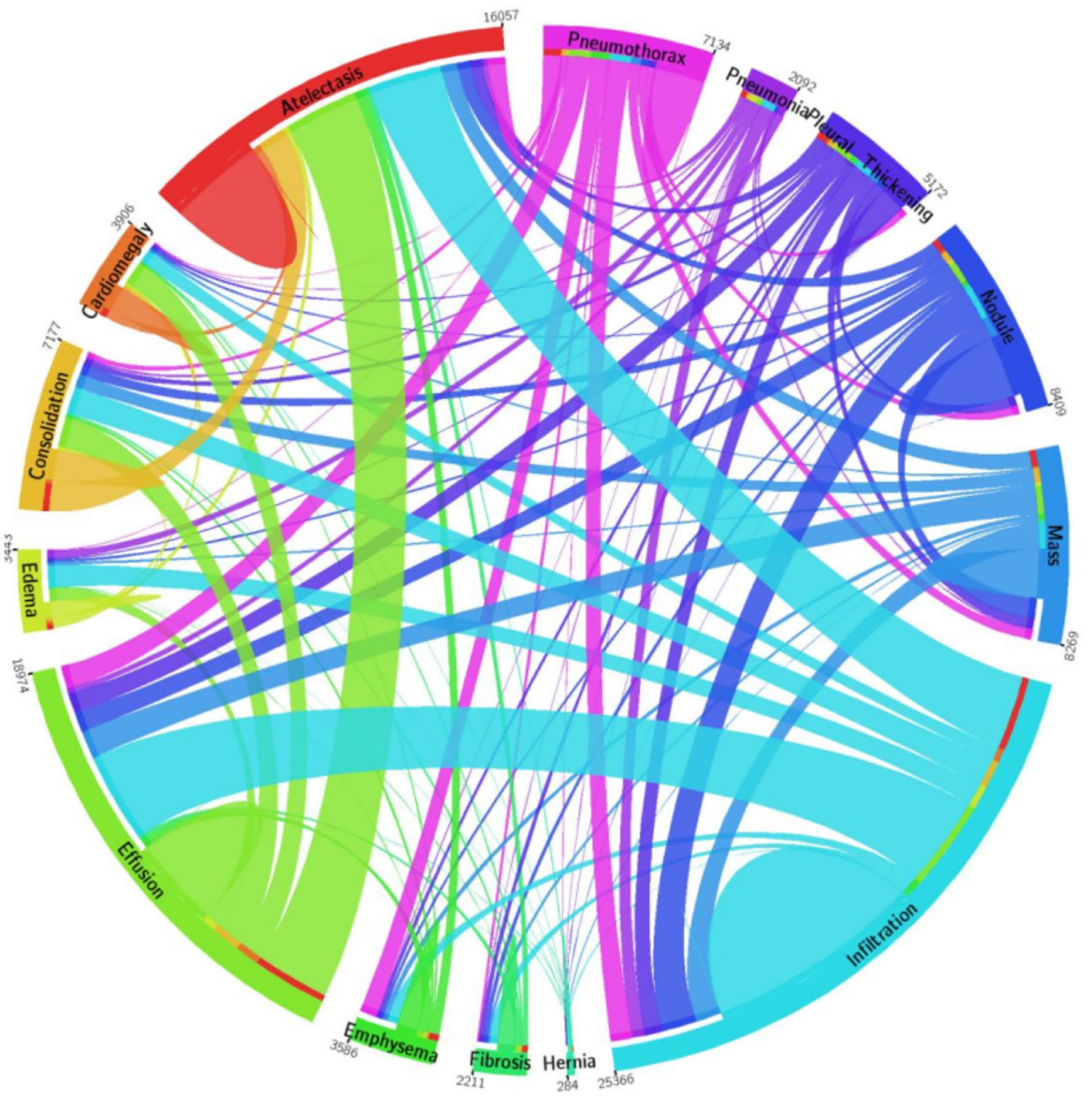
A pictorial view of sample information from the NIH Chest X-ray dataset (47).
